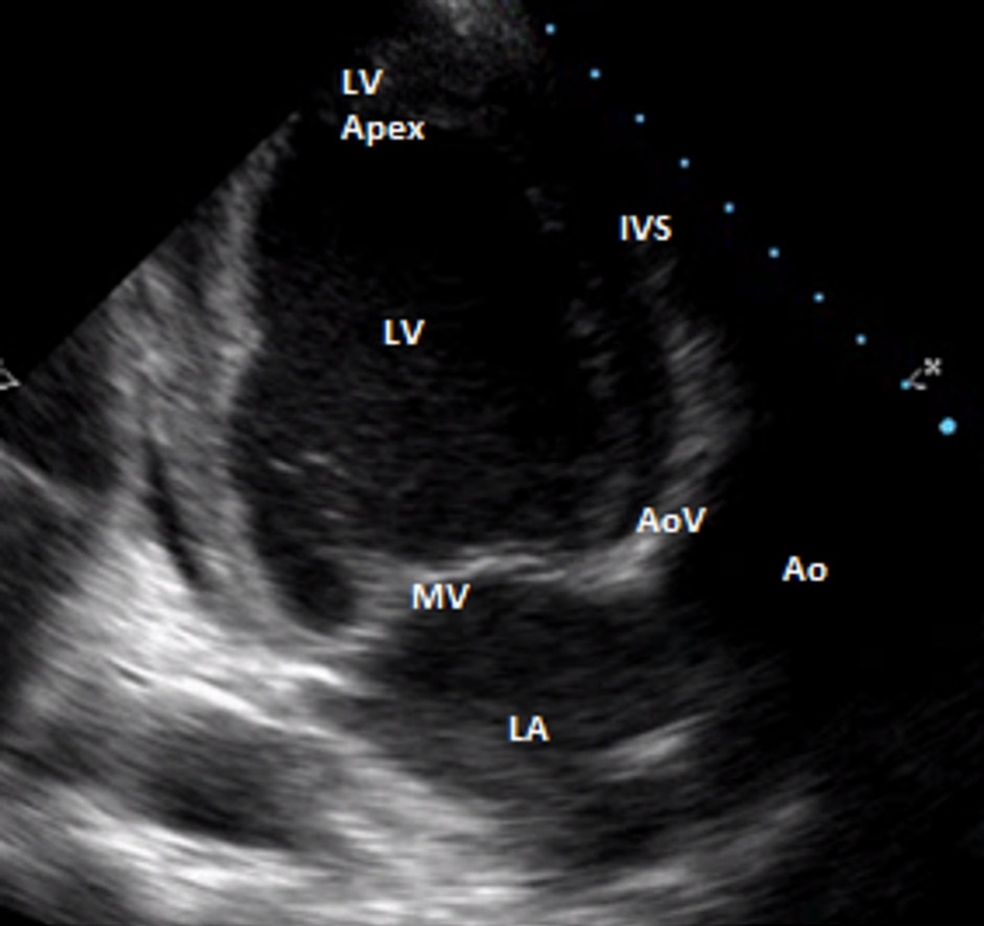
Echocardiogram showing parasternal long axis view. LV: left ventricle; IVS: interventricular septum; Ao: aorta; AoV: aortic valve; MV: mitral valve; LA: left atrium
